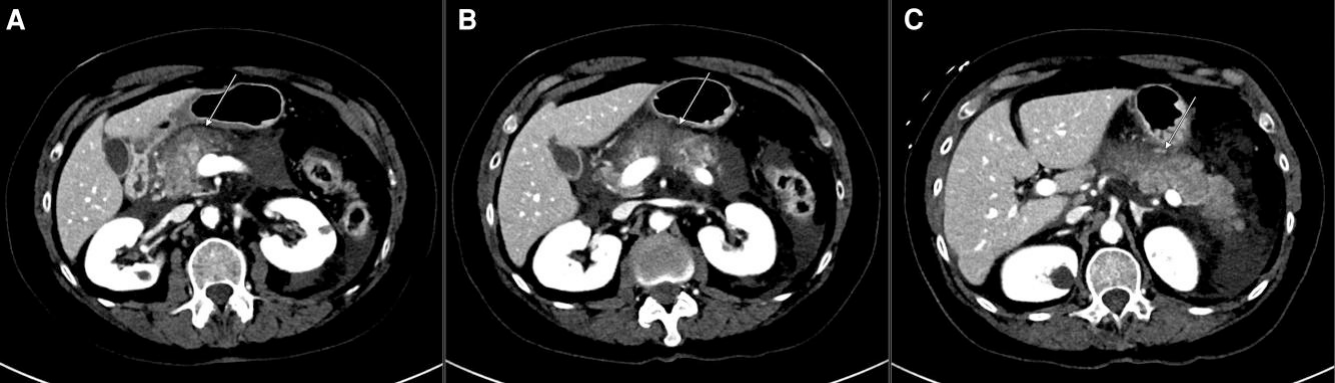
(A-C) Computed tomography scan of the abdomen and pelvis showing heterogeneous enhancement of the pancreatic body with areas of significantly decreased enhancement suggestive of acute pancreatitis with probable partial necrosis of the pancreatic body. There is associated peripancreatic and bilateral paracolic fluid. Arrows pointing to the pancreas.
